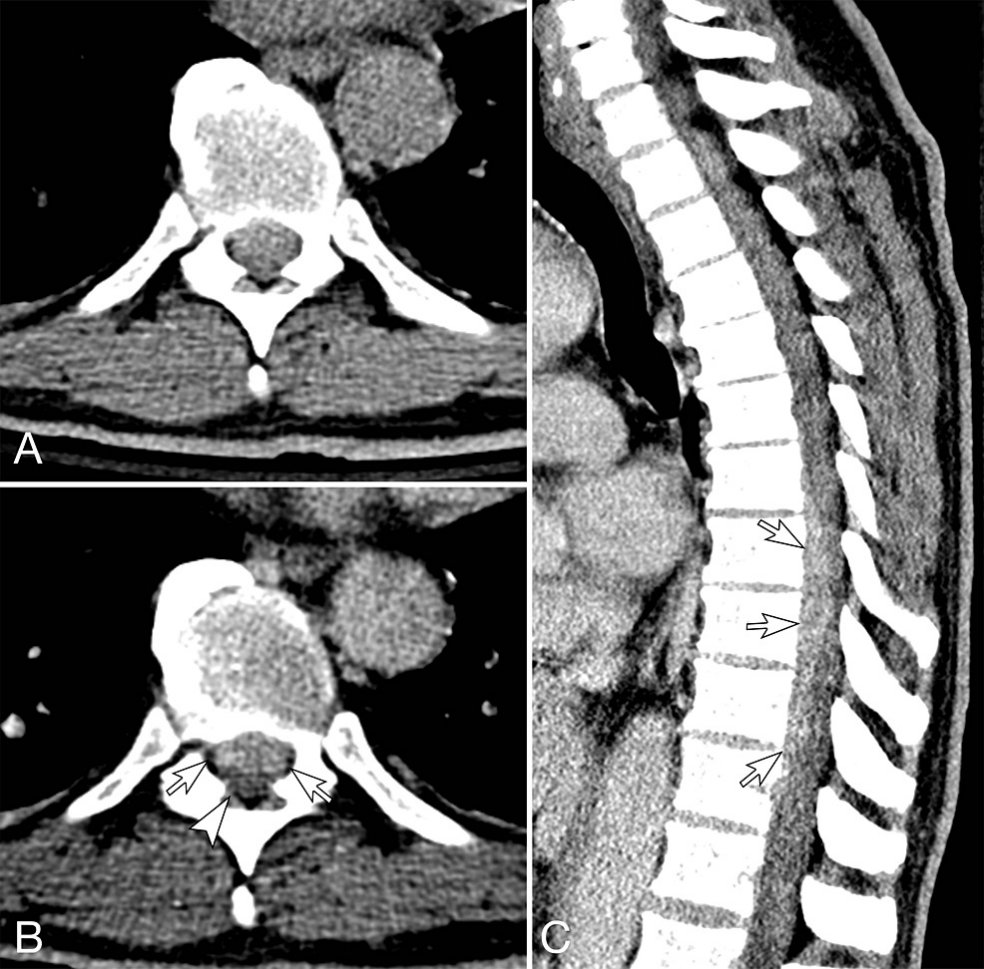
Initial CT of the thoracic spine. Axial pre-contrast (A) and post-contrast (B) and sagittal post-contrast (C) CT images of the thoracic spine. The axial images are at T8-9. In the pre-contrast image (A), it is difficult to identify an abnormality in the spinal canal. In post-contrast images, an enhancing lesion is in the anterior aspect of the spinal canal, spanning from T7 to T11 (white arrows in B-C). Although precise intraspinal location is difficult to determine; this appears to be extradural (not intrathecal), causing a mass effect on the thecal sac and spinal cord (black arrow in B).
